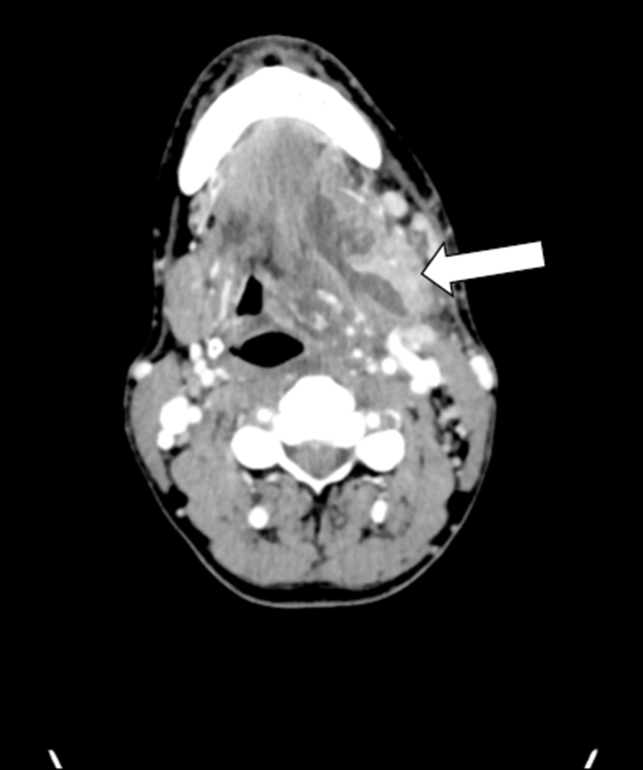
Axial contrast-enhanced computed tomography of the neck revealing a 4.7 cm multiloculated fluid collection (arrow) within the left submandibular space, with surrounding edema, inflammation, and gas collection.
